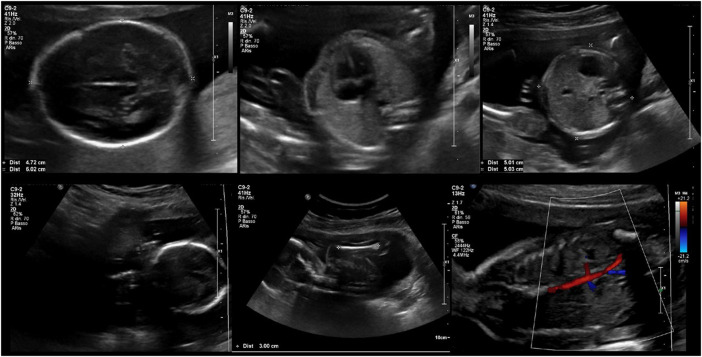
Fetal ultrasound assessment at 20 + 4 weeks of gestation.
